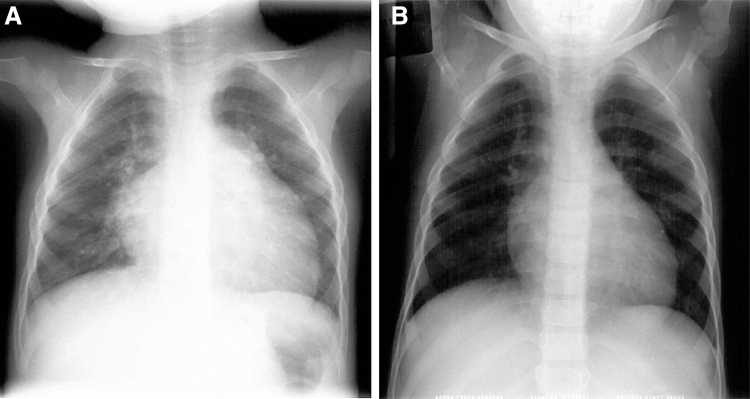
Thorax X-ray of patient 9, table 1, comparing the initial findings (21 months old, with suprasystemic PP, positive answer to the O_2_Test, and hyperreactivity of pulmonary vasculature), with current findings (19 years old). (A) Initial thorax X-ray: important cardiomegaly with enlargement of right atrium and right ventricle and important dilation of the pulmonary trunk and central branches. (B) current thorax X-ray after 17 years of follow-up (FC 1): There is mild enlargement of the right atrium and right ventricle. FC, functional class.
